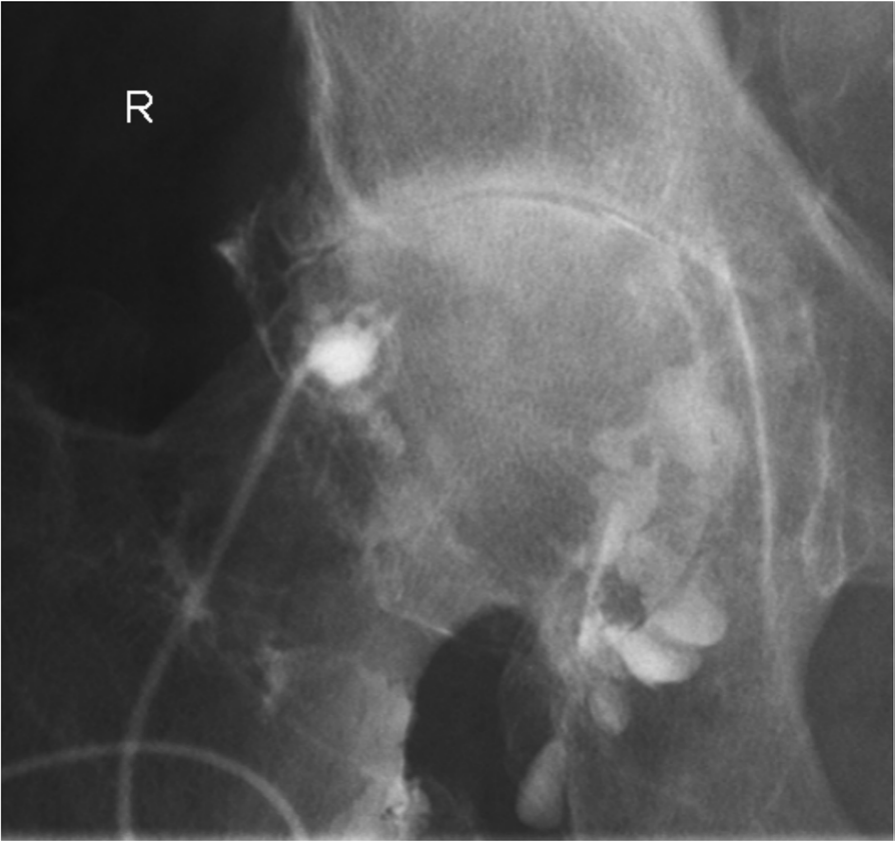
Radiographs of injection and hip arthroplasty. The anteroposterior x-ray shows how contrast agent is injected into the hip joint through a direct anterior approach to the right hip
